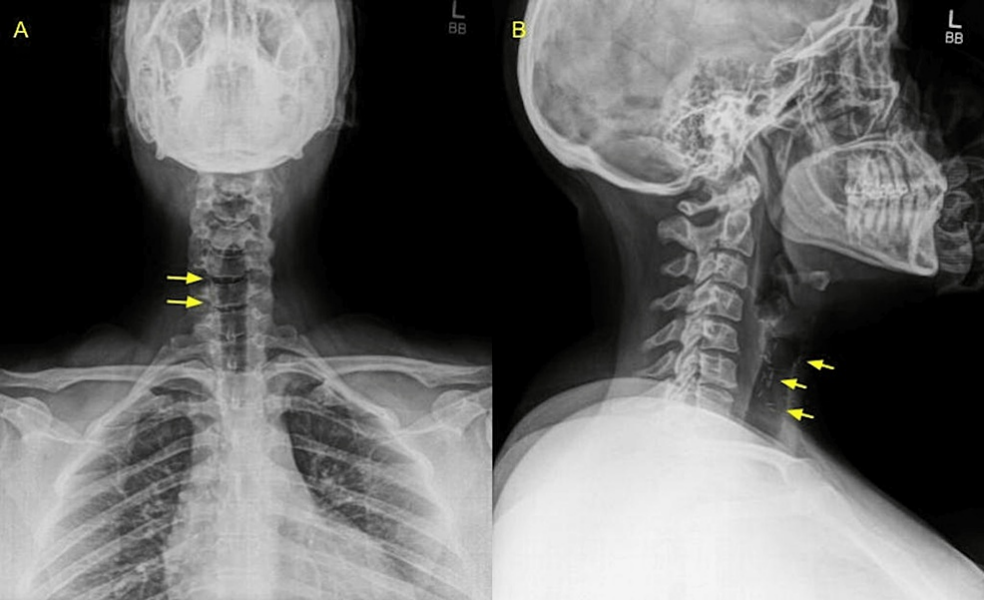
Cervical X-ray with frontal (A) and lateral (B) views of the neck showing surgical clips (yellow arrows).
